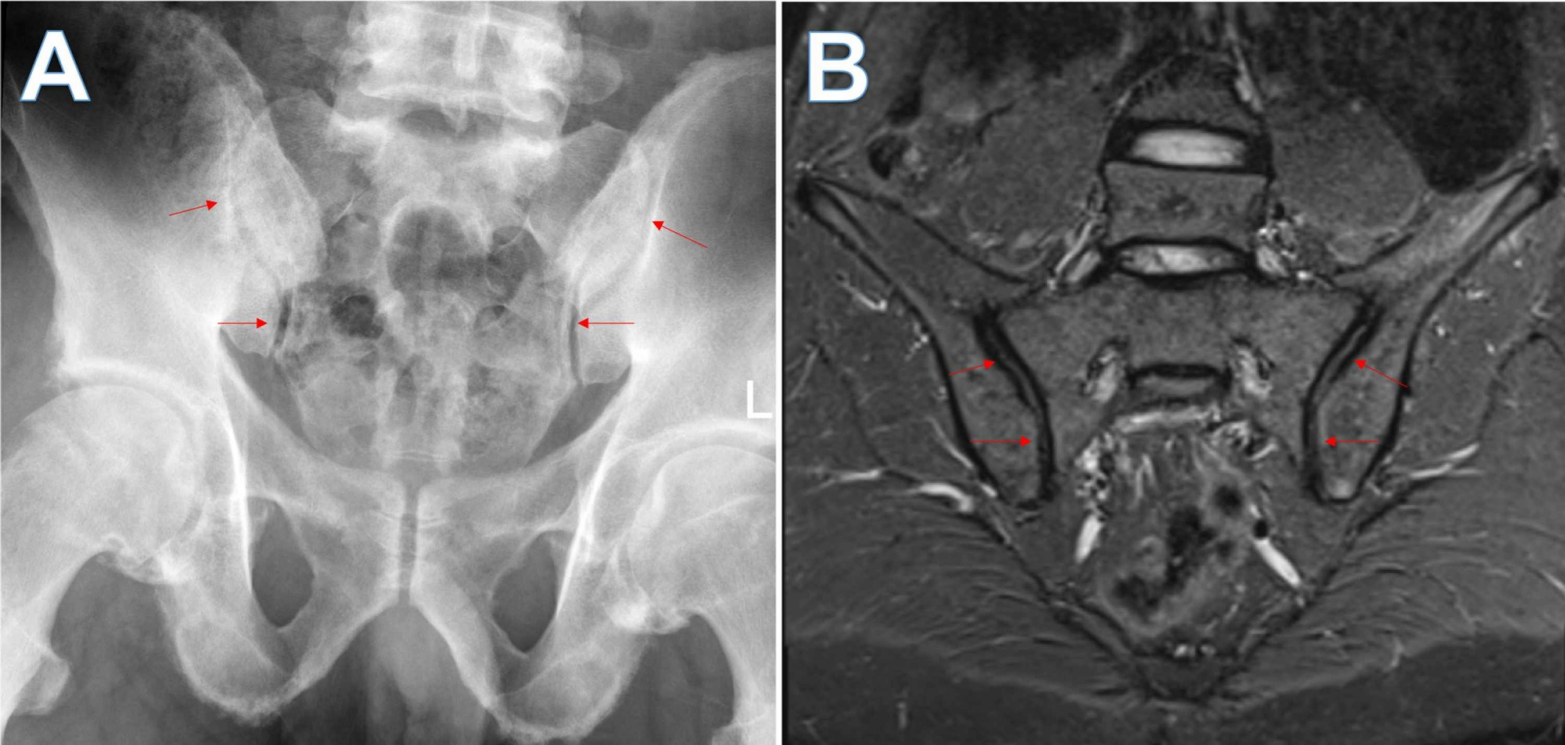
(A) Radiograph and (B) coronal T2 weighted fat suppressed inversion recovery MRI shows normal sacroiliac joints bilaterally (arrows)
